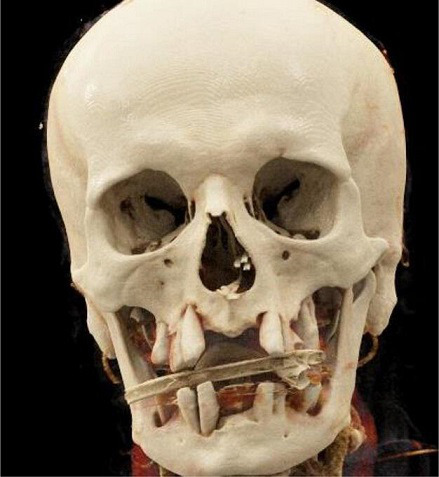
Volume Rendering Technique of cranial CT scan demonstrating alveolar bone loss and significant dental degradation.
